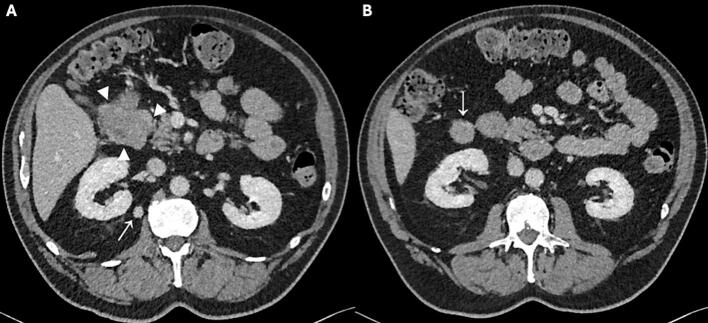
Portal venous phase abdominal CT in axial direction, showing a mass (between arrowheads in A) in the proximal duodenum with a maximum diameter of 56 mm and slight enhancement. Besides, two nodular lesions at the anterior and medial fascias of the right kidney are present (arrows in A and B).
